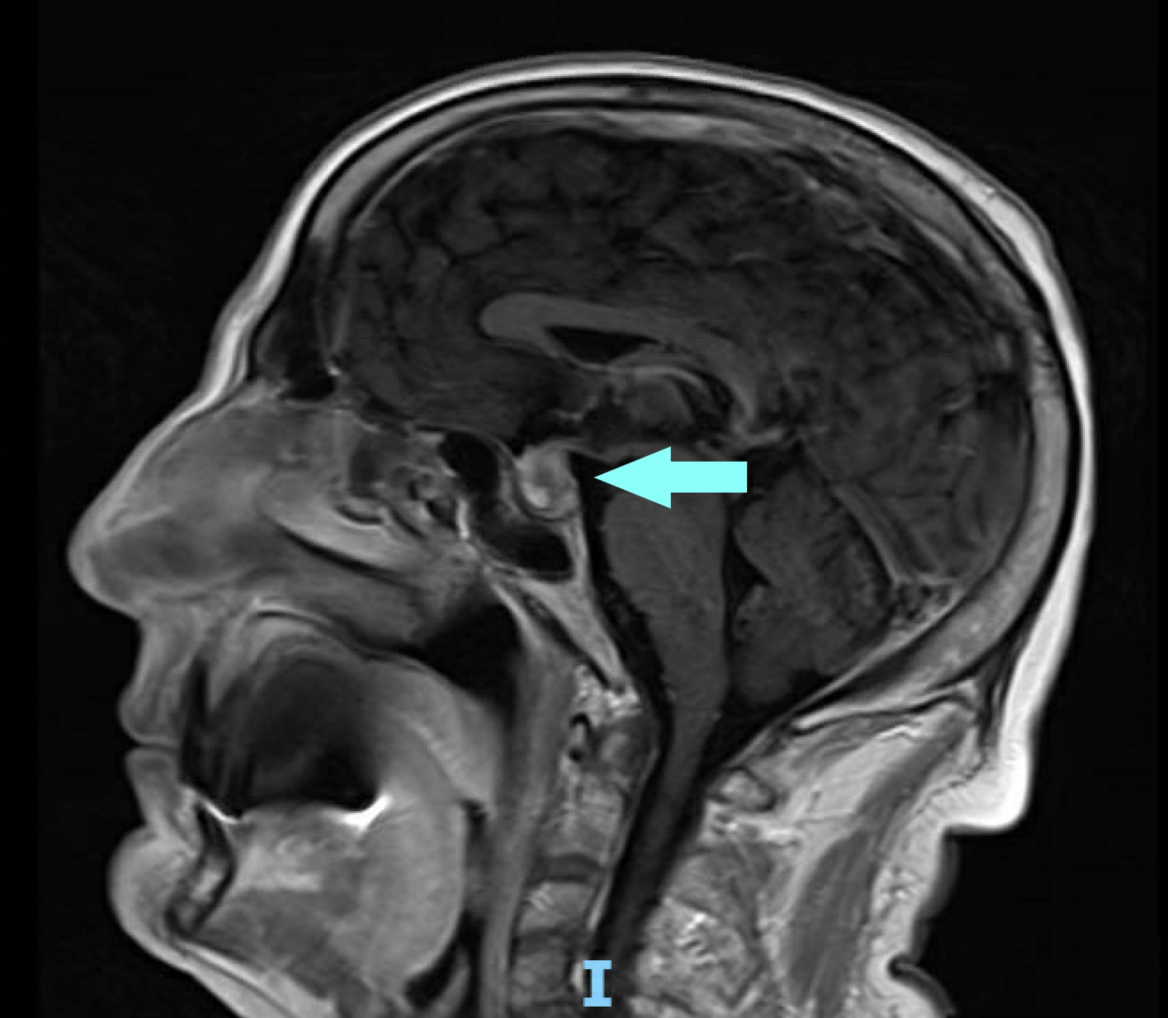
Magnetic resonance imaging showing pituitary macroadenoma in sagittal view
